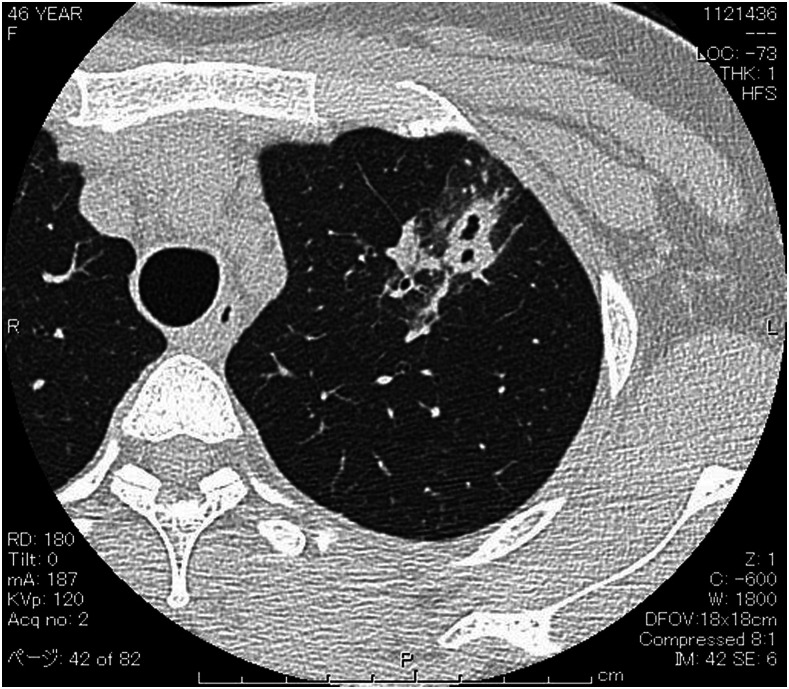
Chest computed tomography.
